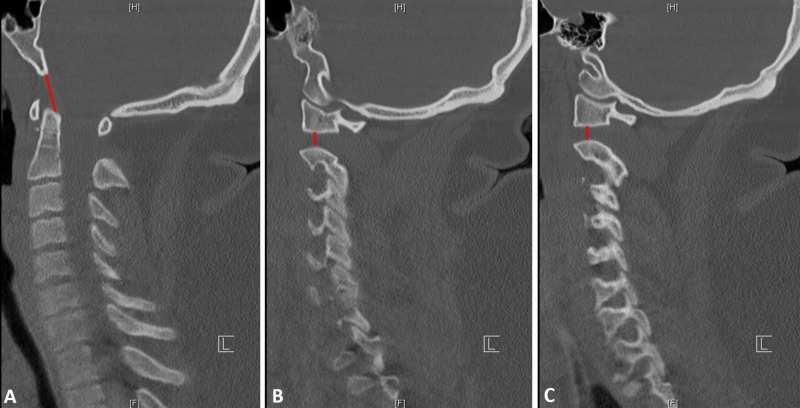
Atlanto-occipital dissociation Sagittal (A) and bilateral parasagittal (B and C) computed tomography (CT) scans showing atlanto-occiptial dissociation diagnosed by the basion interval index > 12 mm (red line A) and atlanto-axial dislocation with increased distraction of the atlanto-axial joints bilaterally (red lines B and C). Note that the condyle-C1 (CCI) interval was normal (B and C).
